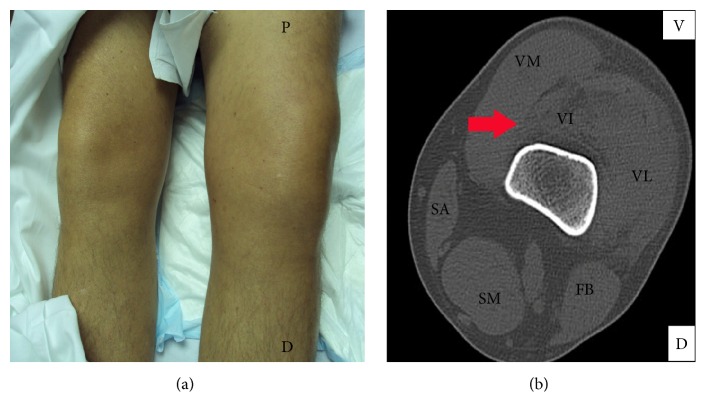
(a) Asymmetry can be observed between the two limbs due to swelling in the left thigh. P: proximal. D: distal. (b) The CT scan shows the presence of a perifemoral haematoma (indicated by the red arrow). The haematoma affects the vastus intermedius (VI) and a small image of active bleeding is visible in its interior. V: ventral. D: dorsal. VM: vastus medialis. VL: vastus lateralis. VI: vastus intermedius. SA: sartorius. FB: femoral biceps. SM: semimembranosus muscle.
